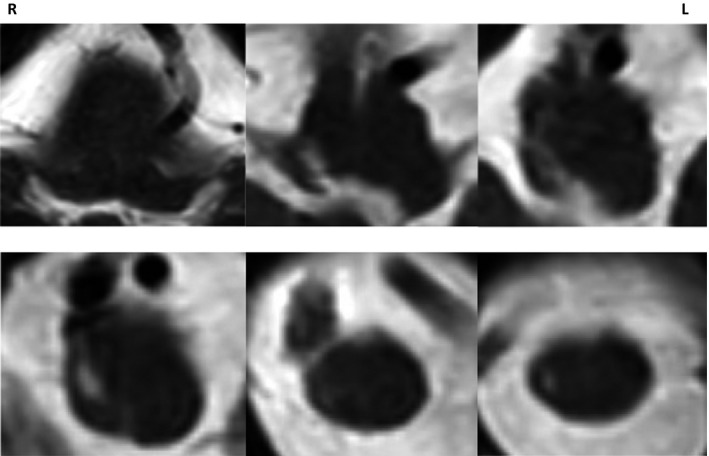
Magnetic resonance T2‐weighted image (MRI‐T2WI) of the medulla oblongata and the upper cervical cord
